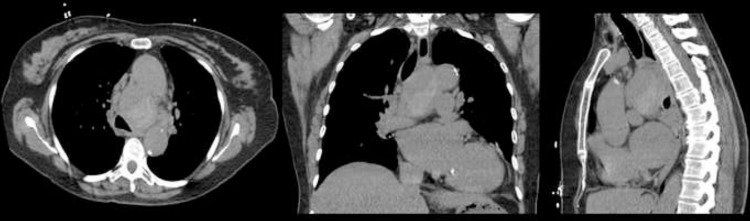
CT chest without contrast demonstrates a middle mediastinal, smooth, round mass measuring 4.2 x 5.5 x 6.7 cm, with internal areas of increased attenuation. This was reported as a soft tissue mass concerning for malignancy.
